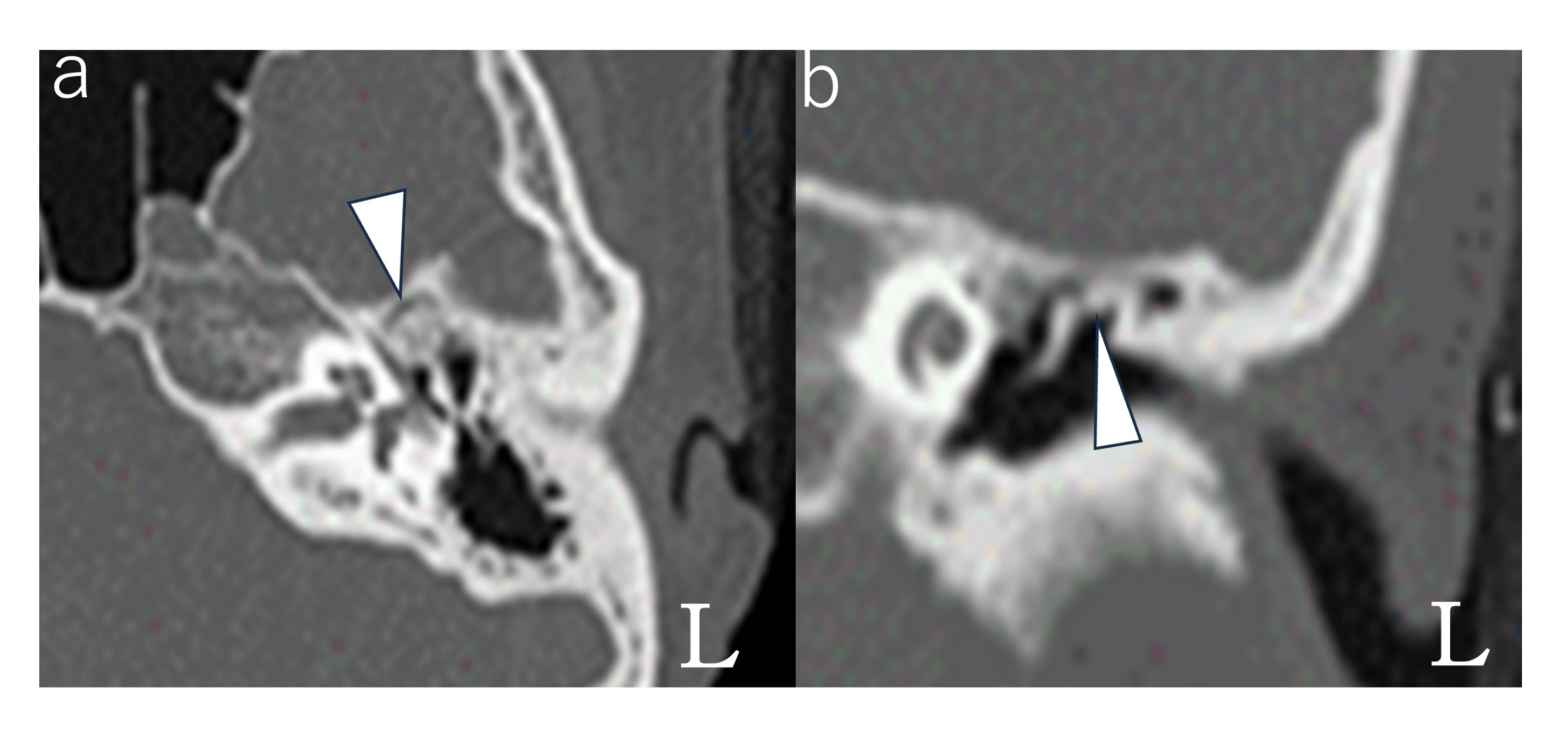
Computed tomography of the left temporal bone An axial computed tomographic image (a) showed a spherical bony lesion occupying a supratubal recess (arrow). A coronal computed tomographic image (b) showed a bony lesion extending from the tympanic canopy to the incus bone (arrow).
